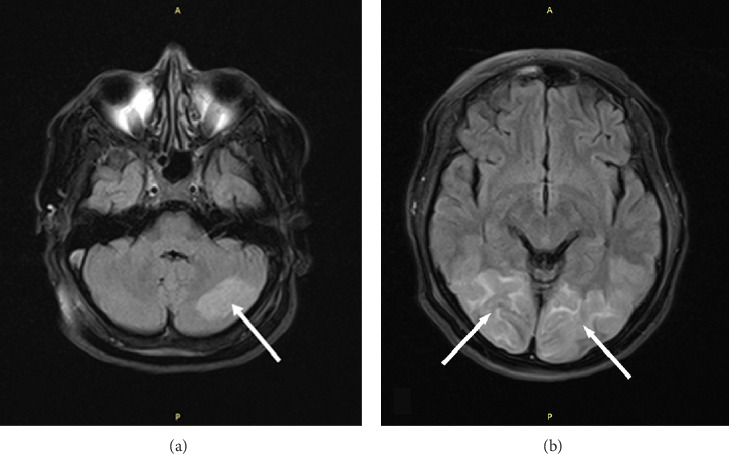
FLAIR sequence screening showed increased signal intensity in the left cerebellar hemisphere ((a) white arrow) and posterior occipital regions ((b) white arrows).
